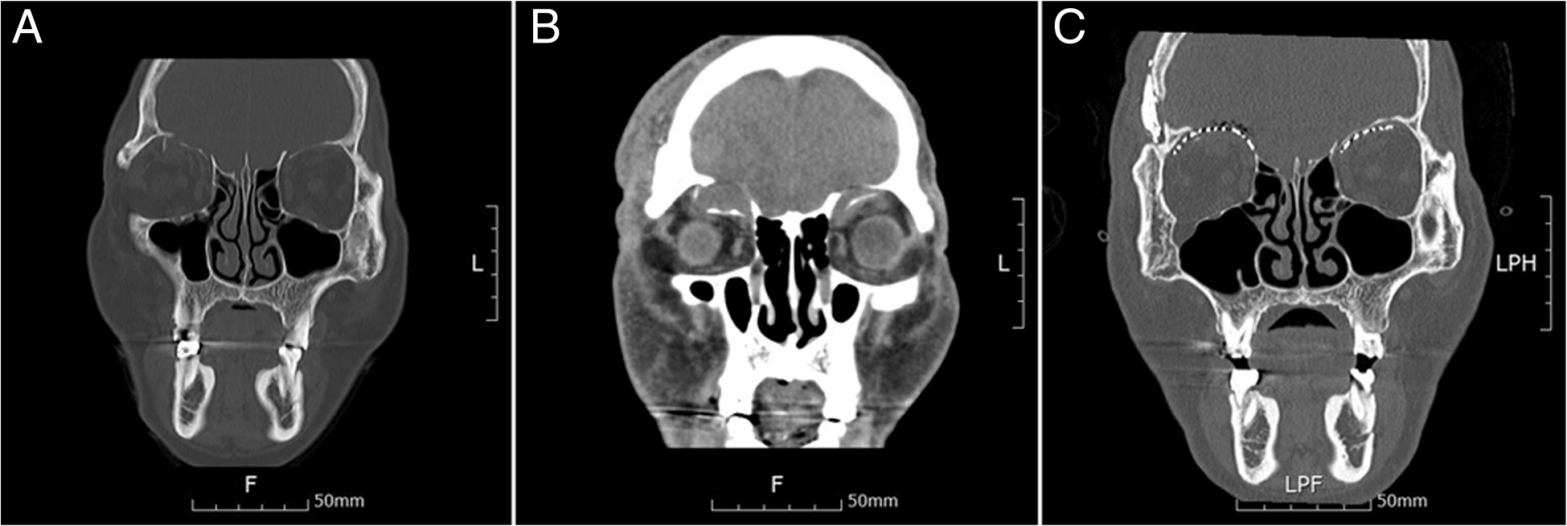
a The displacement of the fracture fragment does not appear severe on computed tomographic (CT) images taken immediately after the injury. b CT scan after the onset of diplopia shows severe displacement of the fracture fragment. c Reconstruction is successful, as confirmed by postoperative CT
